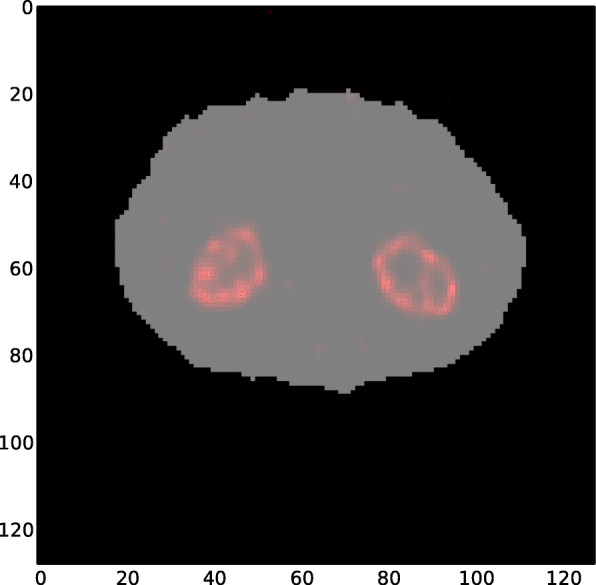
Attenuation map (shown in gray) and reconstructed SPECT image (kidney region displayed only, shown in red) of a representative patient, superimposed
